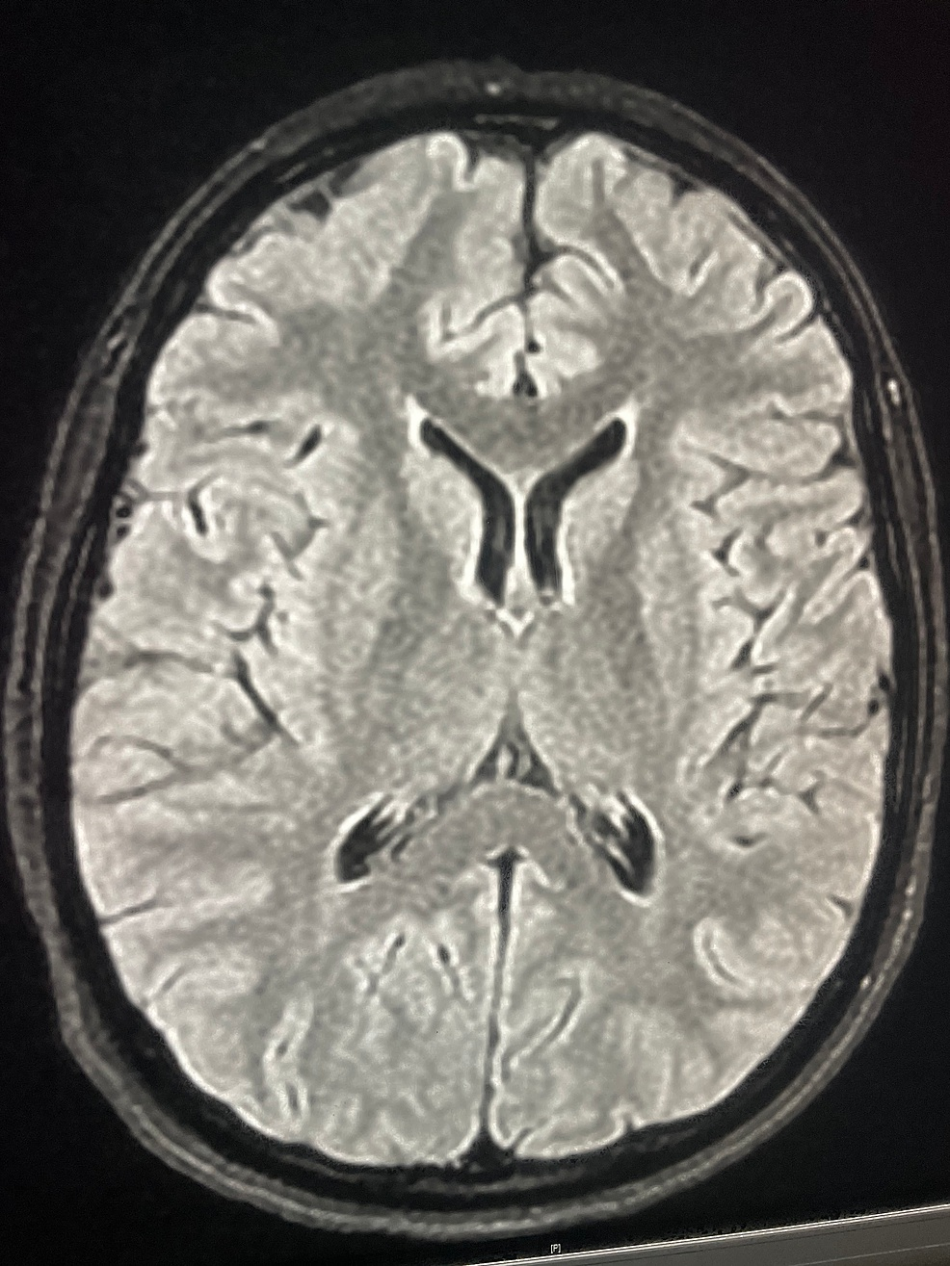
Brain magnetic resonance imaging performed one month after the dual antibiotic therapy (axial section, fluid-attenuated inversion recovery (FLAIR)). FLAIR shows the complete disappearance of the splenial lesion.
